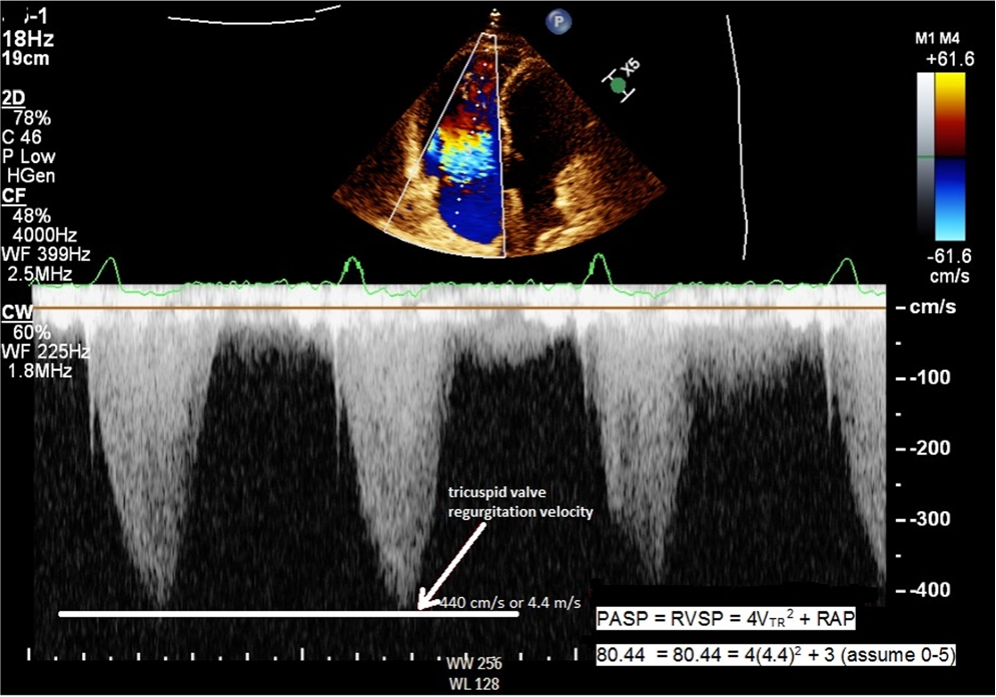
Pulmonary artery systolic pressure (PASP) calculated from a tricuspid jet on echocardiogram. Estimation of PASP utilizing the tricuspid regurgitation jet velocity can be performed according the depicted equation where RVSP is right ventricle systolic pressure and RAP is right atrial pressure which is the same as Jugular venous pressure
